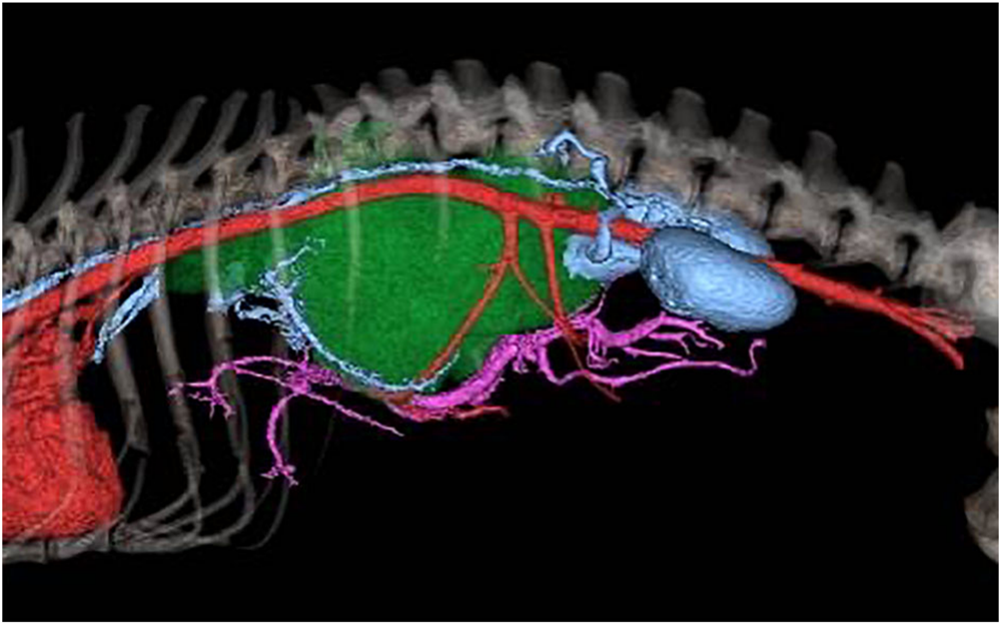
Preoperative three‐dimensional computed tomography image. A large tumour arising from the right adrenal gland fills the caudal vena cava. It was presumed that the interruption of the blood flow caused the formation of a collateral vessel to the azygos vein.
